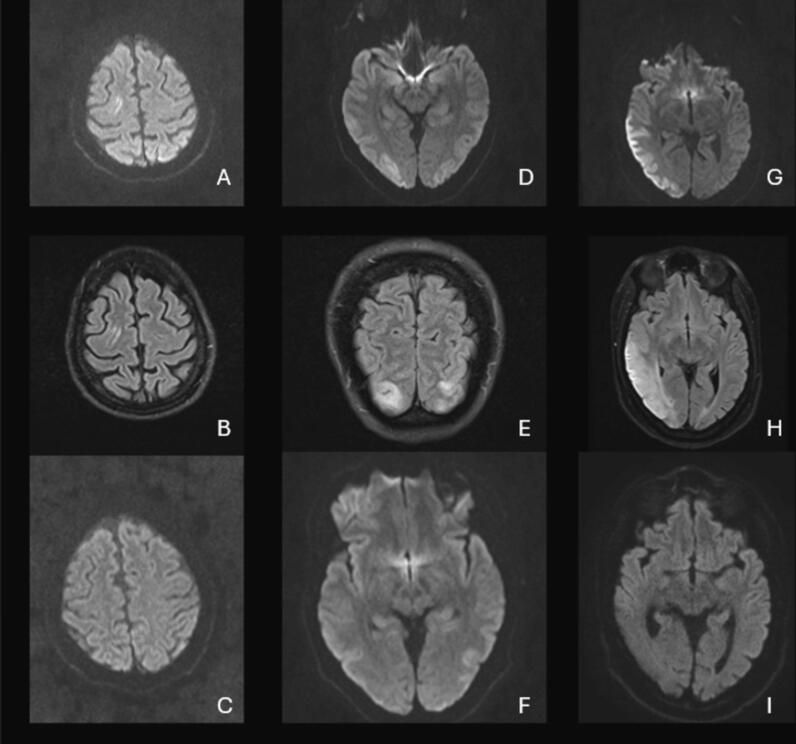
(A) Axial DWI showing diffusion restriction of the right frontal lobe, (B) axial FLAIR showing hyperintensity on the right frontal lobe and (C) axial DWI showing no diffusion restriction four weeks after effective treatment of the first patient with focal status epilepticus and COQ8A-related CoQ10 deficiency presenting with myoclonia. (D) Axial DWI showing bilateral temporooccipital diffusion restriction, (E) coronal FLAIR showing bilateral occipital hyperintensity and (F) axial DWI showing improvement in the diffusion restriction two weeks after clinical improvement of the second patient with COQ8A-related CoQ10 deficiency presenting focal status epilepticus and cortical blindness. (G) Axial DWI showing right temporooccipital diffusion restriction, (H) axial FLAIR showing signal hyperintensity in the same region and (I) axial DWI showing improved findings after treatment of the third patient with COQ8A-related CoQ10 deficiency. DWI: diffusion-weighted image; FLAIR: Fluid Attenuated Inversion Recovery.*
